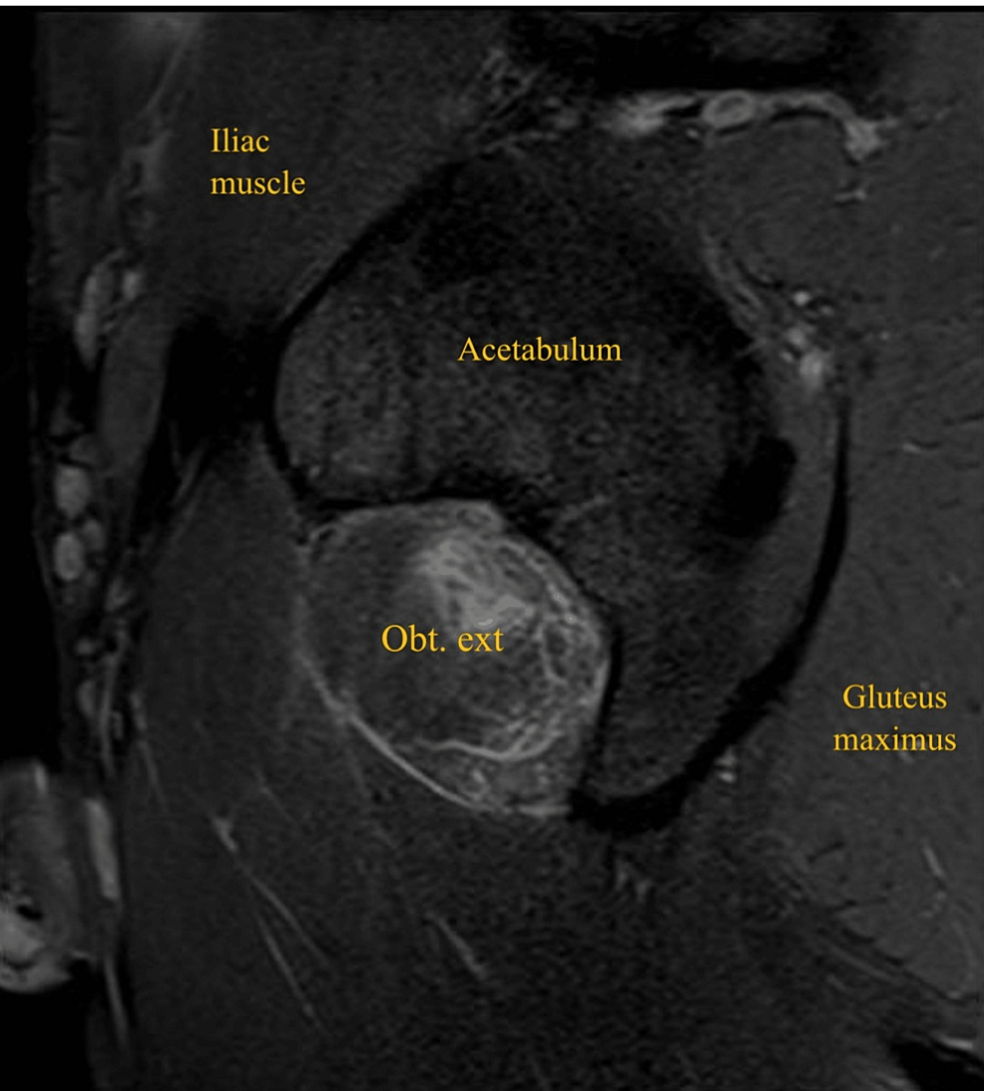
Sagittal short-tau inversion recovery (STIR) MRI of the left thigh showing left obturator externus tear Obt. ext: obturator externus
